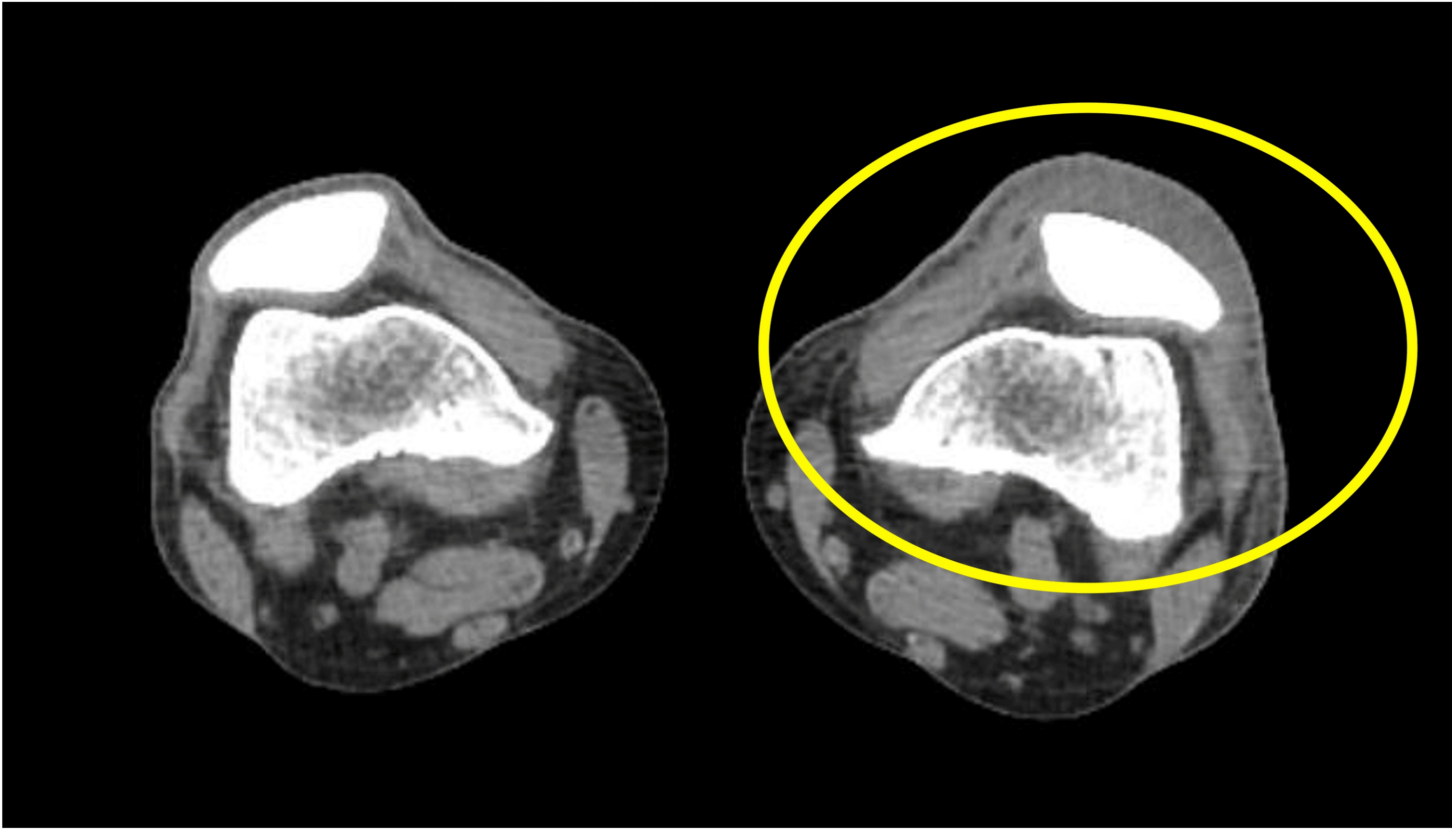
CT scan of the knee, demonstrating increased attenuation of the peri-knee adipose tissue in the left knee (circled in yellow) without any fluid collection or gas formation.
